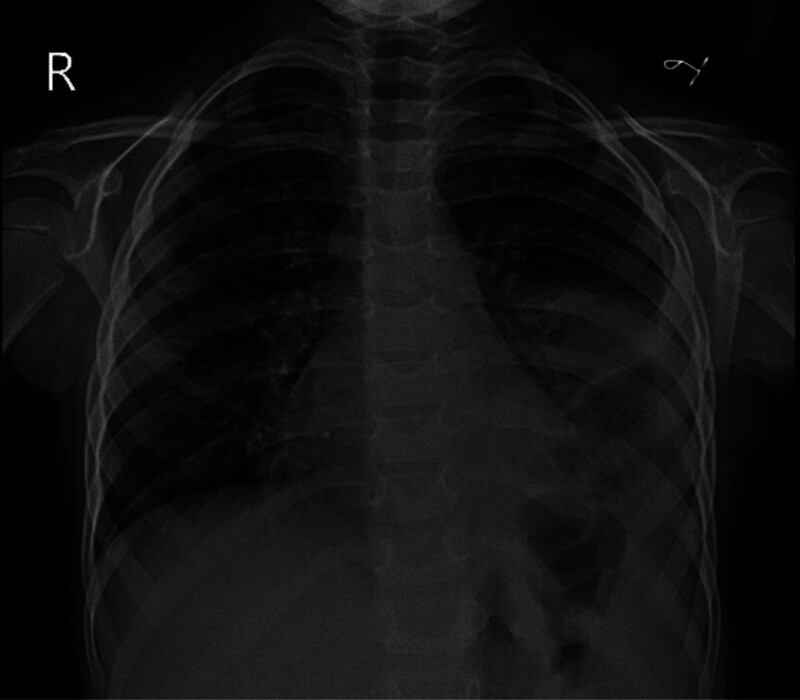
Postoperative chest X-ray (PA view) shows resolution of the previously visualized large cystic lesion. The chest tube has been removed, and a mild residual pleural effusion is noted. No significant abnormalities are observed, and the lung fields appear clear, indicating favorable postsurgical recovery.
